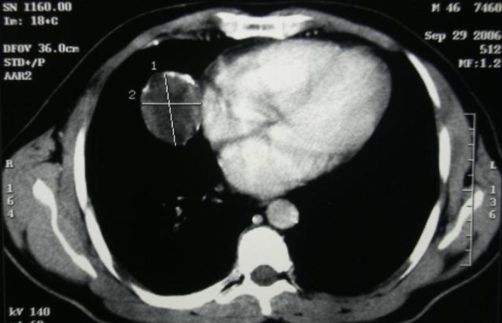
Computed tomography scan showing tissular mass with peripheral calcification in the right lung.
